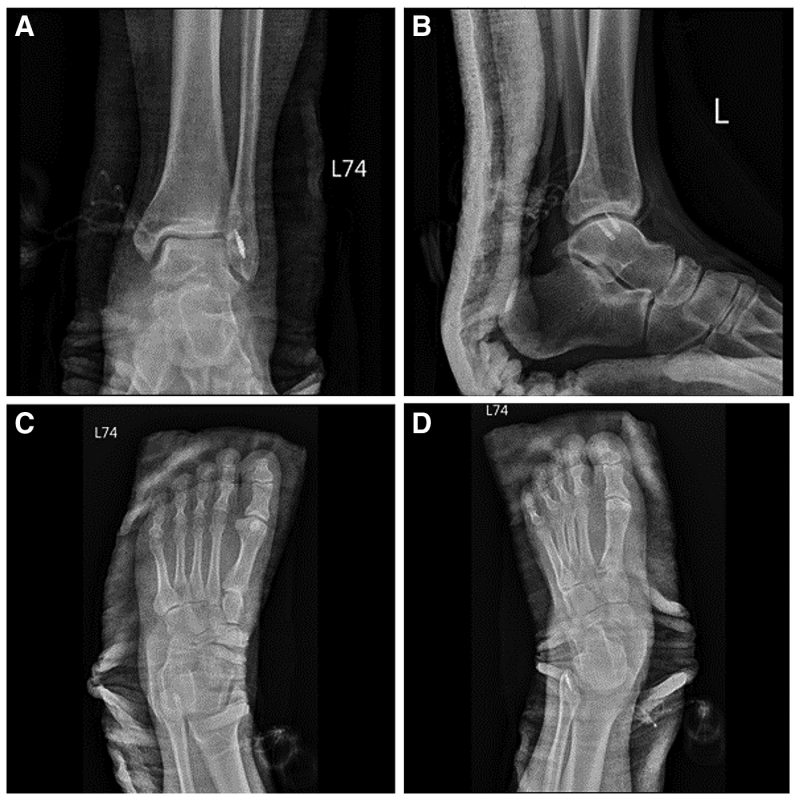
Postoperative plain radiographs of the left ankle and foot. (A) Anteroposterior view and (B) lateral view of the left ankle, and (C) anteroposterior view and (D) lateral view of the left foot, demonstrating appropriate placement and alignment of the navicular osteochondral allograft within the middle concave area. The graft demonstrates close integration with the surrounding bone structures, with no evidence of displacement or gaps. Additionally, 2 suture anchors are visible in the fibula, placed during anterior talofibular ligament repair.
